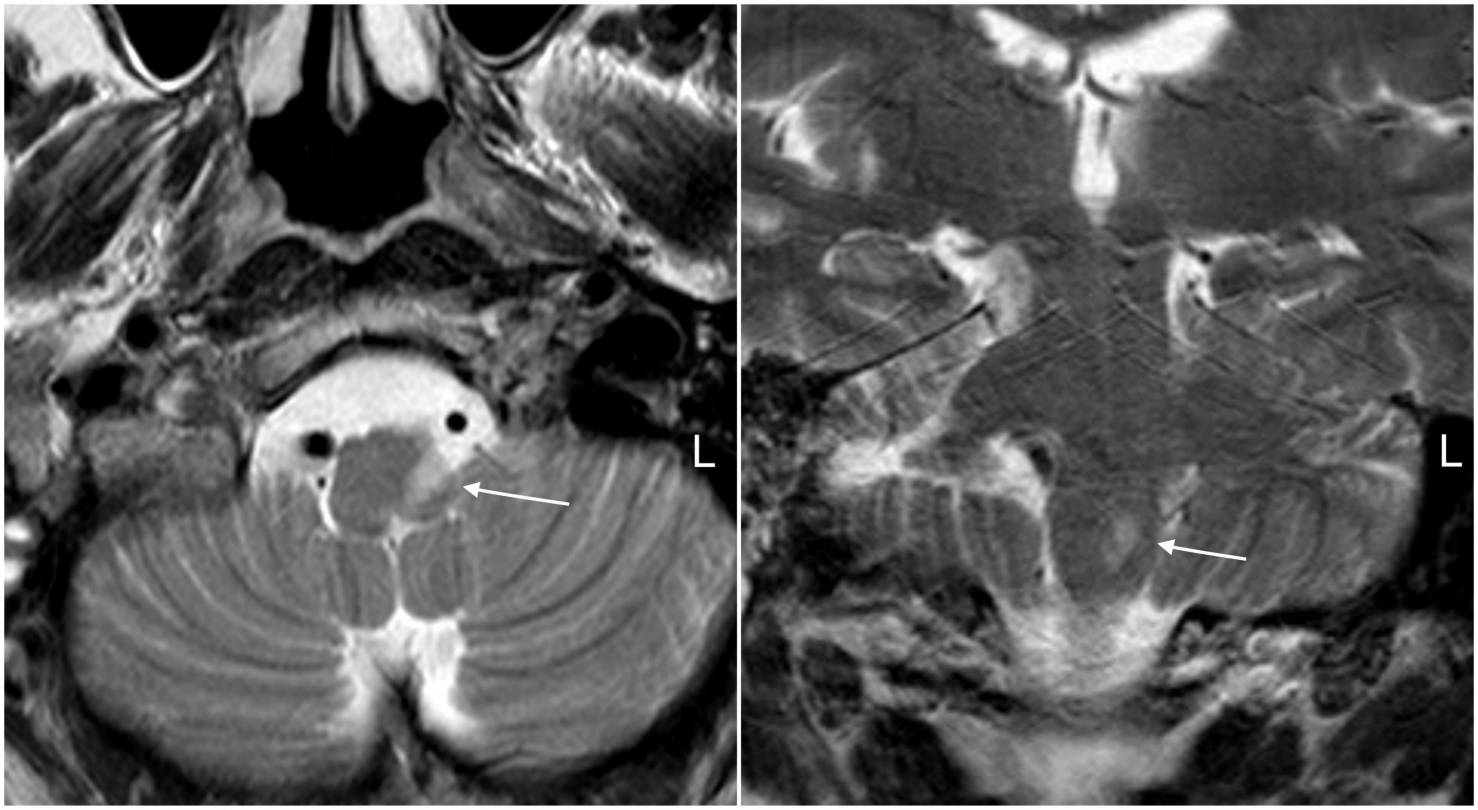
Brain magnetic resonance imaging (MRI) Left: T2 sequence axial MRI displays a hyperintense area located in the left lateral medullary area (arrow). Note the diameter difference between both the vertebral arteries. Right: T2 sequence coronal showing the same finding.
